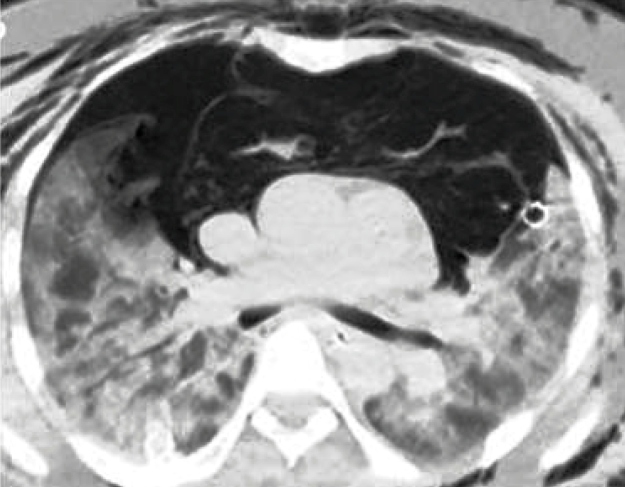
Axial non-enhanced chest computed tomography image revealing diffuse bilateral ground-glass opacities and consolidations in the lungs, pneumomediastinum, bilateral pneumothorax, and excessive subcutaneous emphysema in the chest wall.
